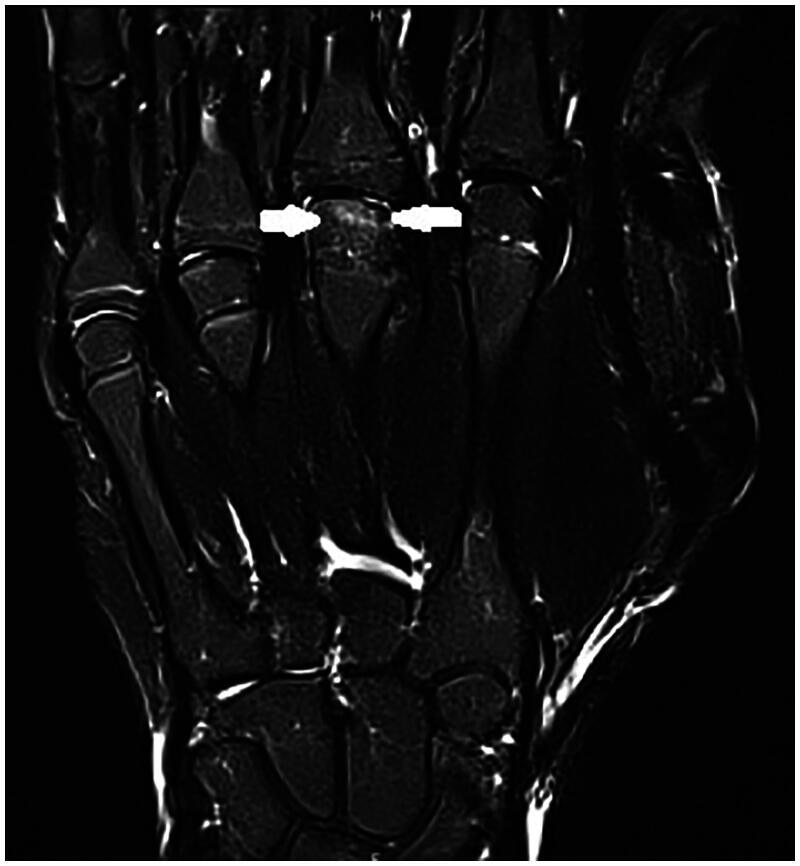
Second MRI, October 2022, vascularization seems preserved in T2 weighted images.
